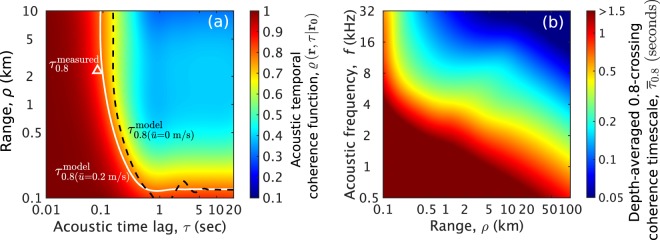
Prediction of acoustic temporal coherence loss caused by traveling surface gravity waves in an isovelocity continental-shelf environment (Fig. 1(a)) at general ranges and frequencies for ocean sensing and communication. A wind speed of 5 m/s (significant waveheight $${H}_{1/3}=0.56$$ m and WMO sea state 3) is used. Both effects of surface waveheight decorrelation and Doppler spread from horizontal surface wave motion accumulate with increasing range and reduce acoustic coherence by more than 20% within seconds. Doppler effects become important at high frequencies for underwater communication and fine-scale sensing. (a) Acoustic temporal coherence function, $$\varrho \left({\bf{r}},\tau | {{\bf{r}}}_{{\bf{0}}}\right)$$ (Eq. (3)), predicted as a function of range and acoustic time lag at 17 kHz and 18 m receiver depth. The horizontal mean particle velocity at the sea surface in the forward direction $${\bar{u}}=0.2$$ m/s. The white solid line denotes the modeled 0.8-crossing coherence timescale ($${\tau }_{0.8}$$). Similarly, the black dashed line shows the modeled $${\tau }_{0.8}$$, but when Doppler effects are not included, i.e. $${\bar{u}}=0$$. The white open triangle indicates the measured $${\tau }_{0.8}$$^28^. (b) Depth-averaged 0.8-crossing coherence timescale ($${\bar{\tau }}_{0.8}$$) predicted as a function of range and acoustic frequency in the same environment. This timescale shortens with increasing frequency and range, where $${\bar{\tau }}_{0.8} < 1.5$$ seconds is only shown. The region where $${\bar{\tau }}_{0.8} > 1.5$$ seconds denotes frequencies and ranges where $$\varrho \left({\bf{r}},\tau | {{\bf{r}}}_{{\bf{0}}}\right)$$ does not fall below 0.8. At sufficiently low frequencies or close ranges in a calm sea state, $$\varrho \left({\bf{r}},\tau | {{\bf{r}}}_{{\bf{0}}}\right)$$ does not fall below 0.8.
